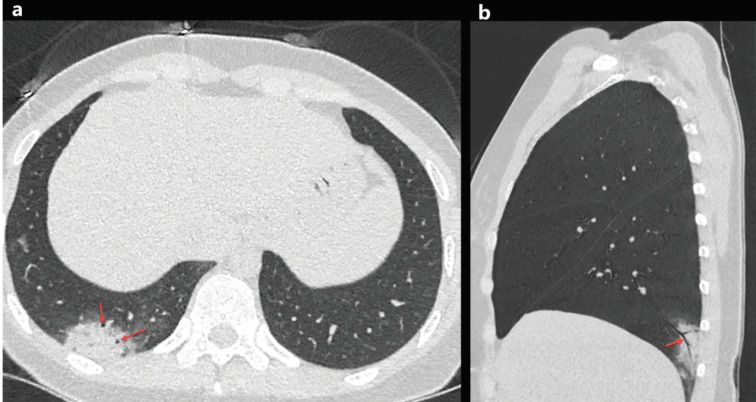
A33-year-old woman with COVID-19 presented with cough, fever, and pleuritic chest pain for 2 days. Axial chest CT image shows a focal consolidation with air bronchogram sign in the right lower lobe (a, red arrows). The sagittal reconstructed CT image of the right lower lobe shows air bronchogram sign inside the consolidation (b, red arrow).
